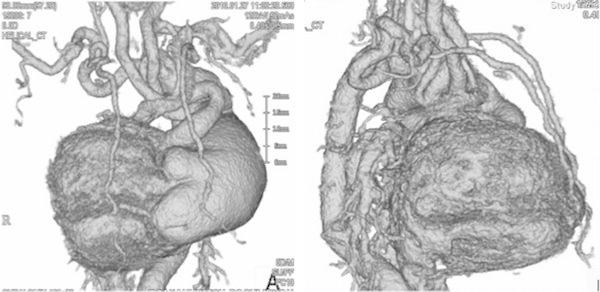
Enhanced computed tomography showed main pulmonary artery aneurysm and tortuosity of the cervical arteries
